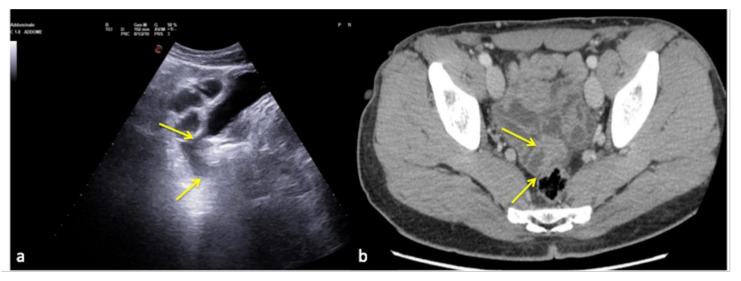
Longitudinal US pelvic scan (a) shows a small, triangular, hypoechoic area which may be misinterpreted as free fluid (arrows). On CT scan (b) it appears to be an intestinal loop (arrows).
